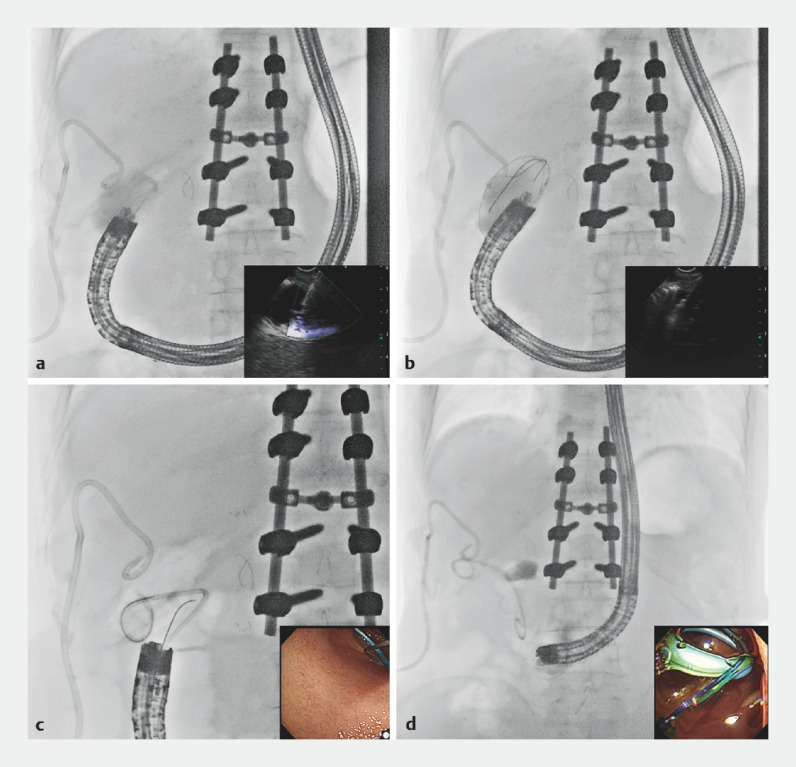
Endoscopic ultrasound-guided gallbladder drainage.
